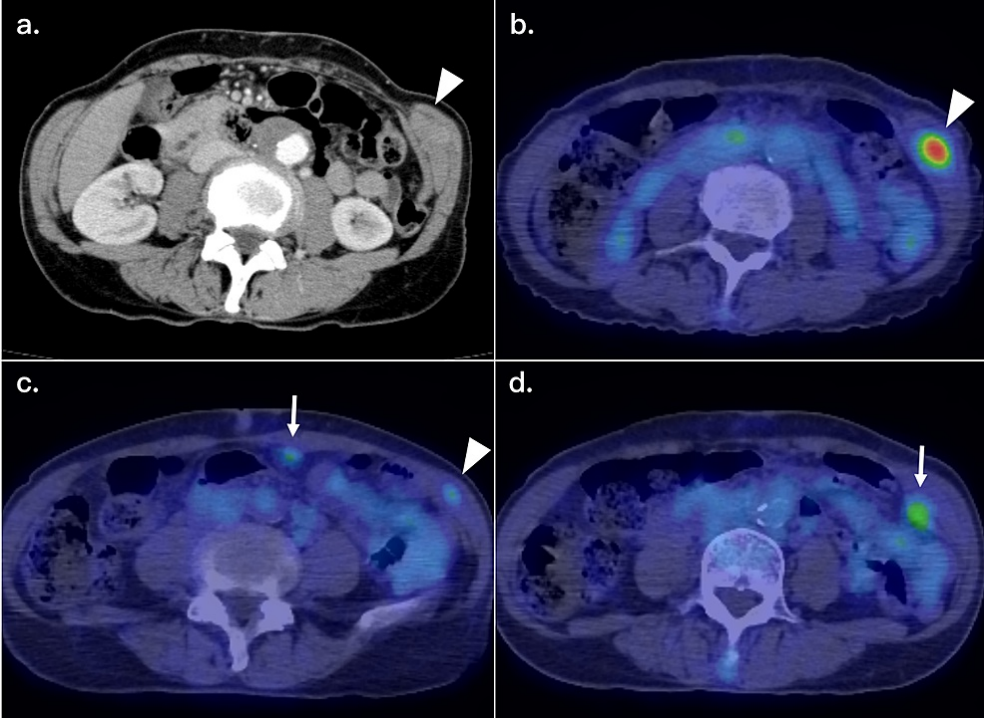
CT and PET-CT findings at the first recurrence (a, b) An enhanced CT scan showed a contrasted nodule in the left abdominal wall, with positive FDG uptake (SUVmax = 6.52). (c, d) PET-CT also showed multiple intraperitoneal and abdominal wall metastases with FDG uptake at the peritoneum (arrows) and in the abdominal wall (arrowheads). CT: Computed tomography; PET-CT: positron emission tomography-computed tomography; FDG: fluorodeoxyglucose; SUVmax: maximum standardized uptake value.
